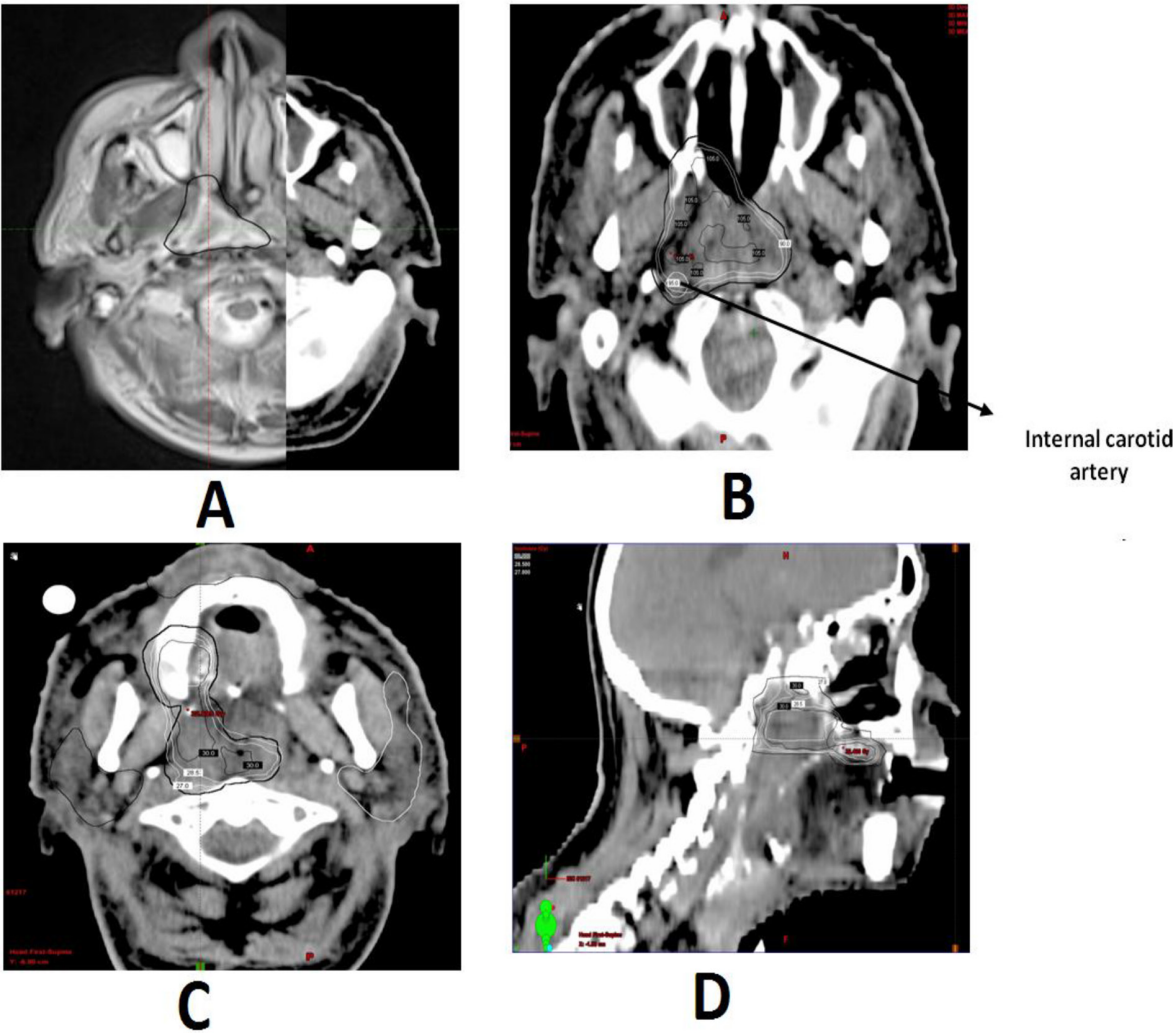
(A) Local recurrence in a patient of carcinoma nasopharynx (diagnosed in February 2011' and treated with chemoradiation) marked in black as delineated on fused CT-MRI scan. Figure 1B, (90%) Isodose with partial sparing of internal carotid artery covering tumor volume for a prescribed dose of 30 Gy/five fractions. Figure 1C,D, Two separate but adjacent GTV's covered in the prescribed dose as shown in axial and saggital views. Patient is alive and remains disease free 27 months after SABR. GTV, gross tumor volume; SABR, stereotactic ablative radiotherapy.
